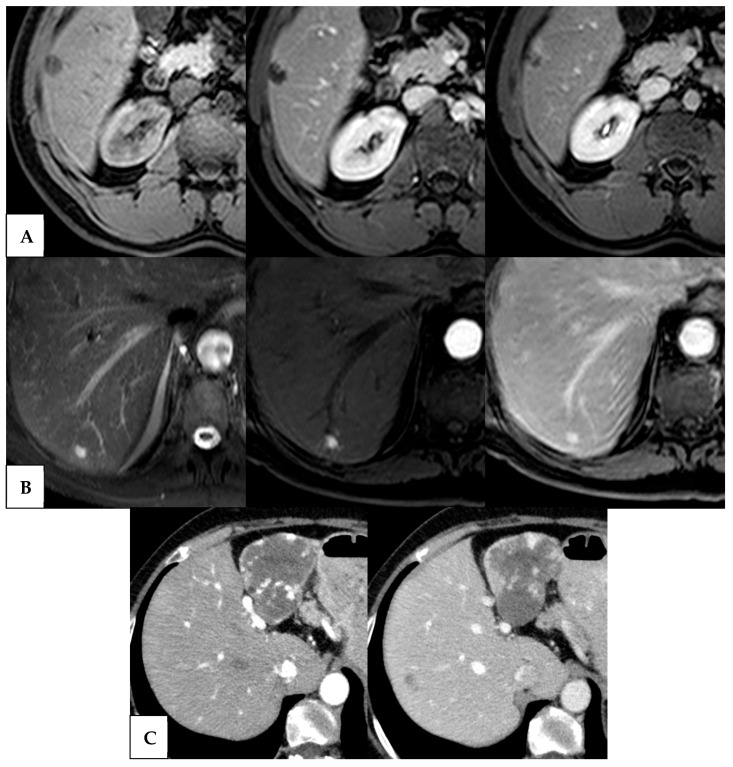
(A) MRI scan showing T2-weighted image and contrast-enhanced T1-weighted images (portal and delayed phases) of a slow-filling hemangioma, (B) MRI scan showing T2-weighted image and contrast-enhanced T1-weighted images (arterial and portal phases) of a flash-filling hemangioma, and (C) CT scan showing arterial and portal phase images of a giant hemangioma.
